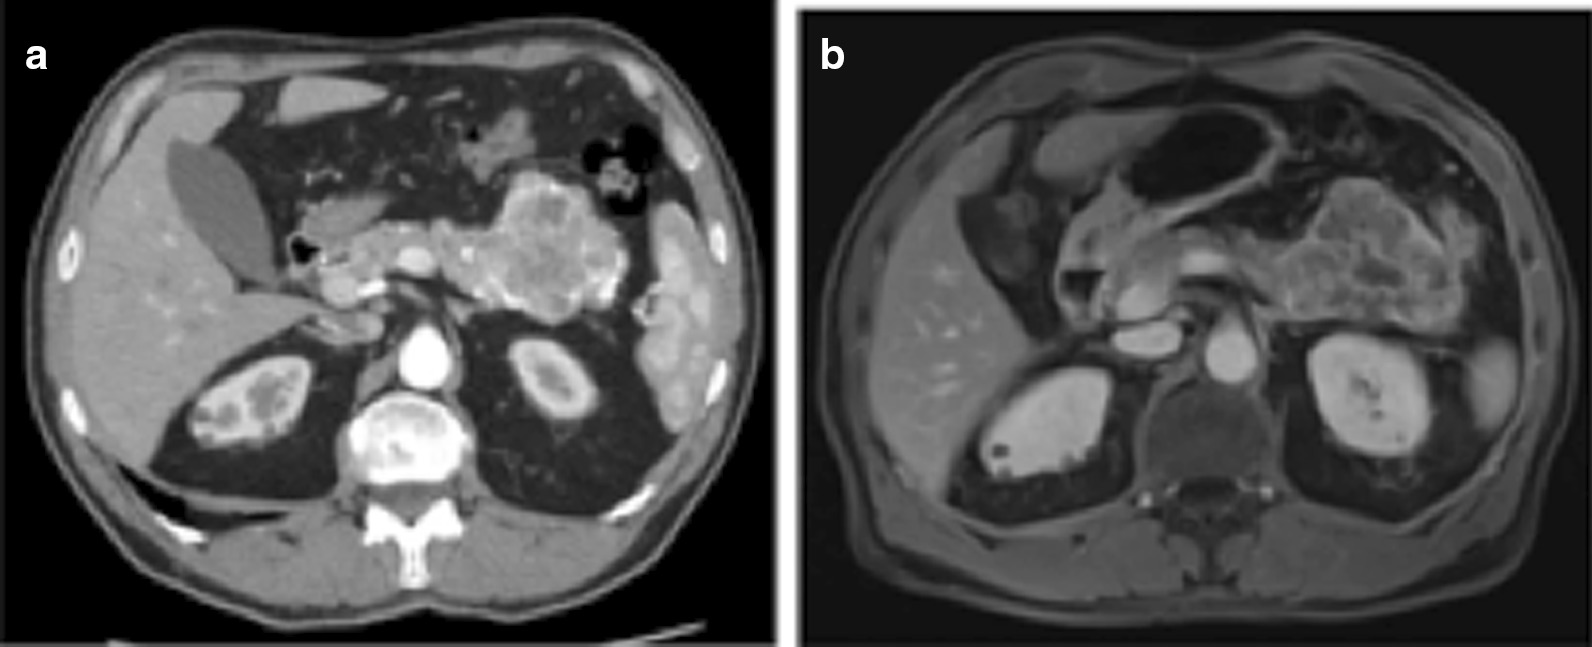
a CT-scan showing a lesion of 7 cm of diameter in the body and tail of the pancreas; b IRM confirming an expansive solid lesion, with multiple septa inside, strictly adherent to the jejunum
